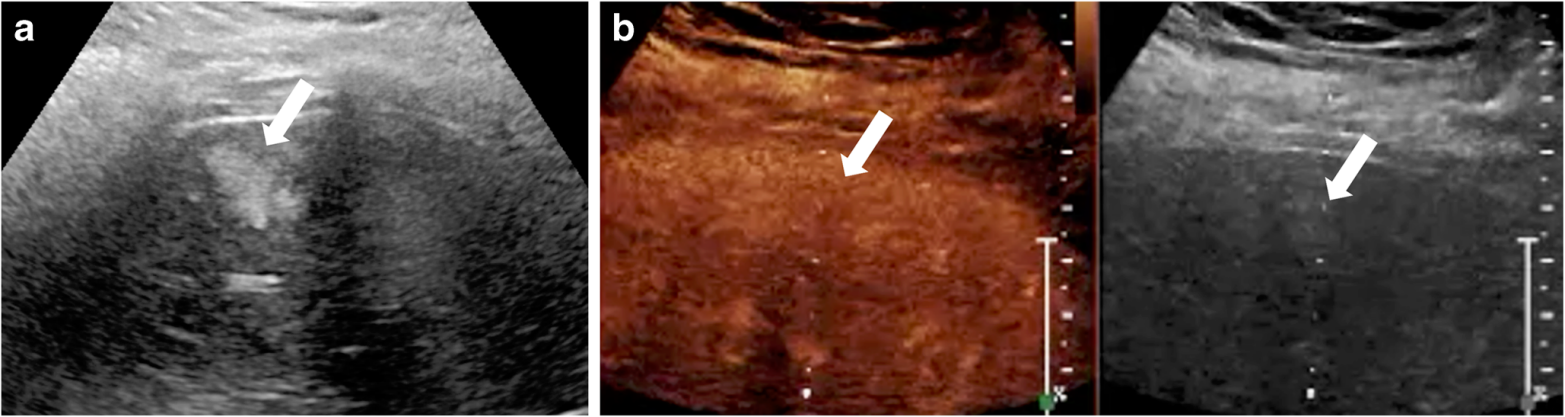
Focal fatty infiltration of the liver in a 16-year-old girl who presented with elevated liver enzymes. a Transverse gray-scale US demonstrates a focal hyperechoic lesion (*arrow*) within the subcapsular region of the liver. b Contrast-enhanced ultrasound (CEUS) of the liver in transverse plane with dual display of gray-scale (*right*) and contrast-enhanced (*left*) images. The lesion (*arrows*) on gray-scale US shows isoenhancement to the liver background on CEUS in the portal venous phase. It was isoenhanced in all other phases, too (not shown)
